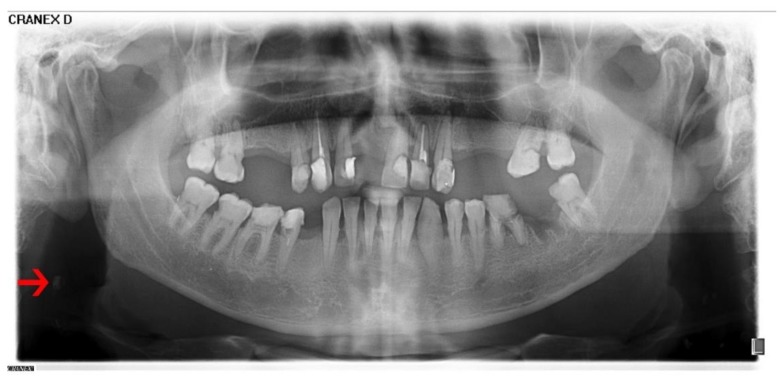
Panoramic radiograph with a detectable carotid artery calcification (red arrow).
